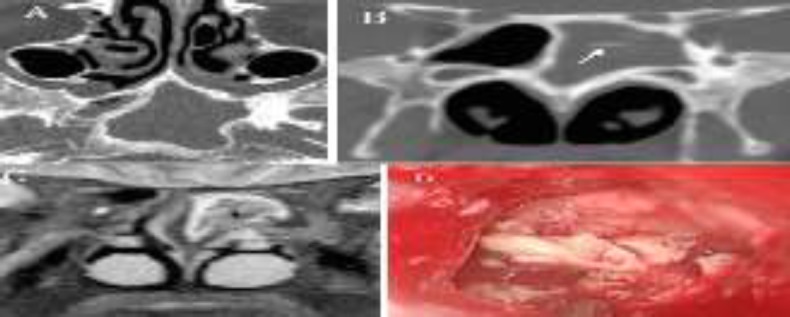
Axial (A) and coronal (B) CT scan demonstrates the opacification of the left sphenoid sinus with calcifications (white arrows) and marked thickening of the walls of the sinus. (C) Coronal T2-weighted MRI indicates an isointense signal from the fungal mass (asterisk) with hyperintense inflamed mucosa at the periphery of the sinus. Intraoperative view of the fungal concrement in the sphenoid sinus floor (D)
